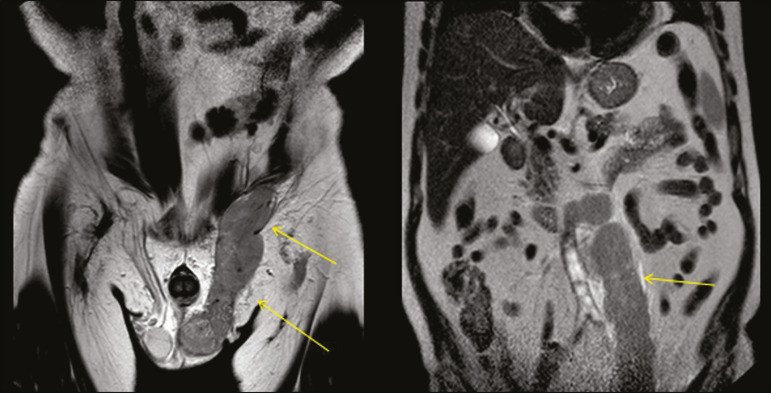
Coronal T2-weighted MRI sequence showing primary testicular lymphoma extending through the inguinal canal and the left gonadal vein (arrows).
